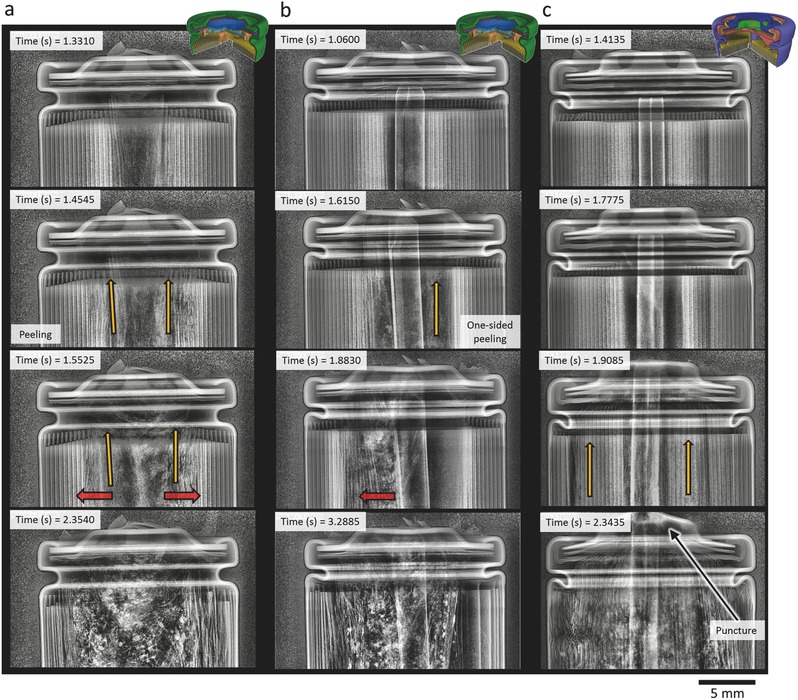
Time‐stamped radiographs of a) LG‐S3, b) LG‐B4, and c) Sanyo cells undergoing thermal runaway. Radiography movies of (a), (b), and, (c) are provided as Movies S1, S3, and S5, respectively, in the Supporting Information. The red arrows highlight the radial direction of propagation of thermal runaway, and the yellow arrows highlight the direction of peeling and shift of the electrode assembly.
